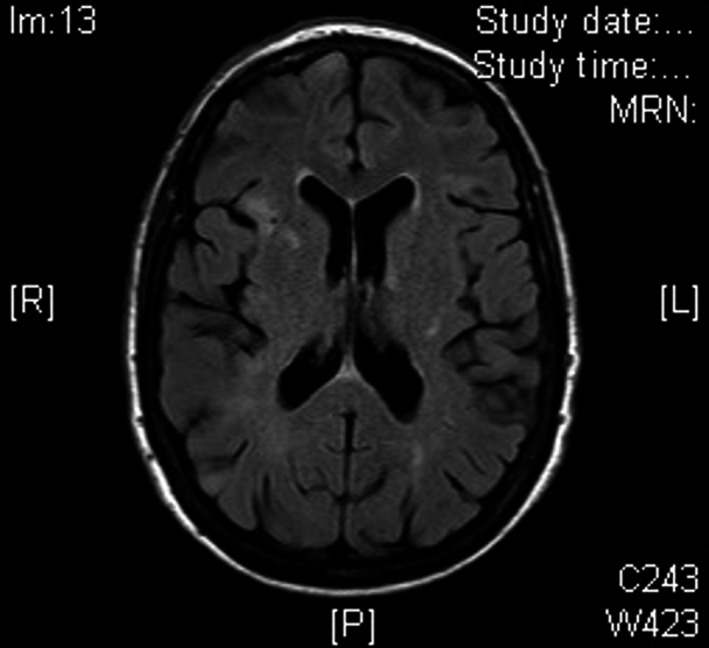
Magnetic resonance imaging (MRI) brain FLAIR sequence showing multiple cortical and subcortical white matter lesions in both cerebral hemispheres consistent with cerebral tubers.
